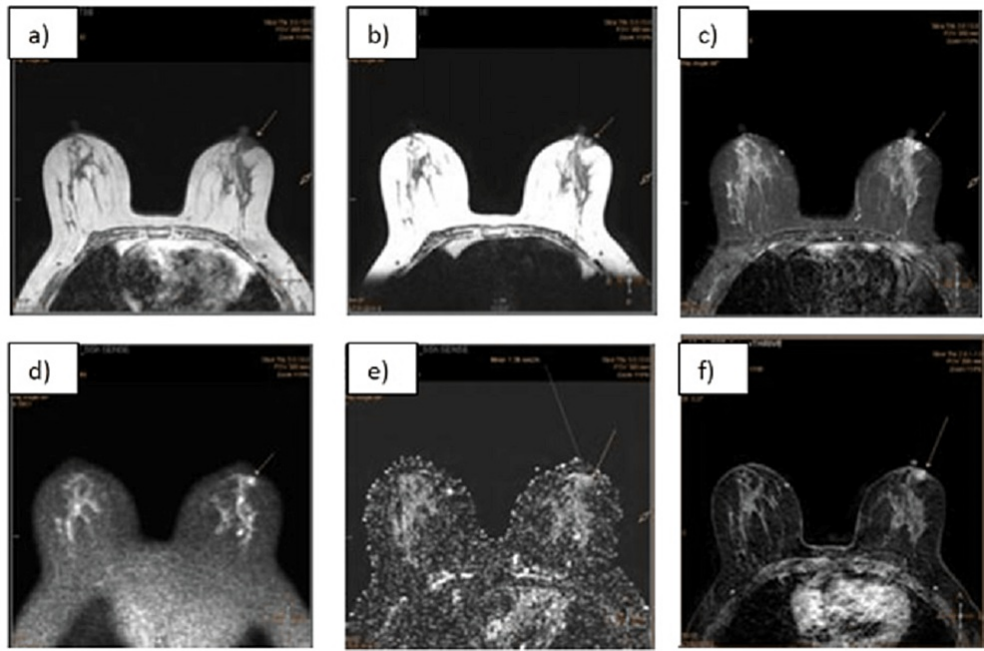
MR mammography: morphological features a) An irregular non-circumscribed mass (marked with an arrow) is showing a hypointense signal on T1WI; b) The lesion shows a hyperintense signal on T2WI; c) The irregular mass shows a hyperintense signal on STIR images in the peri-areolar region of the left breast; (d,e) Patchy diffusion restriction is seen in the lesion on DWI/ADC images (marked with arrow); (f) Heterogeneous avid contrast enhancement is seen in the lesion on post-contrast images STIR: short-tau inversion recovery; DWI: diffusion-weighted imaging; ADC: apparent diffusion coefficient
